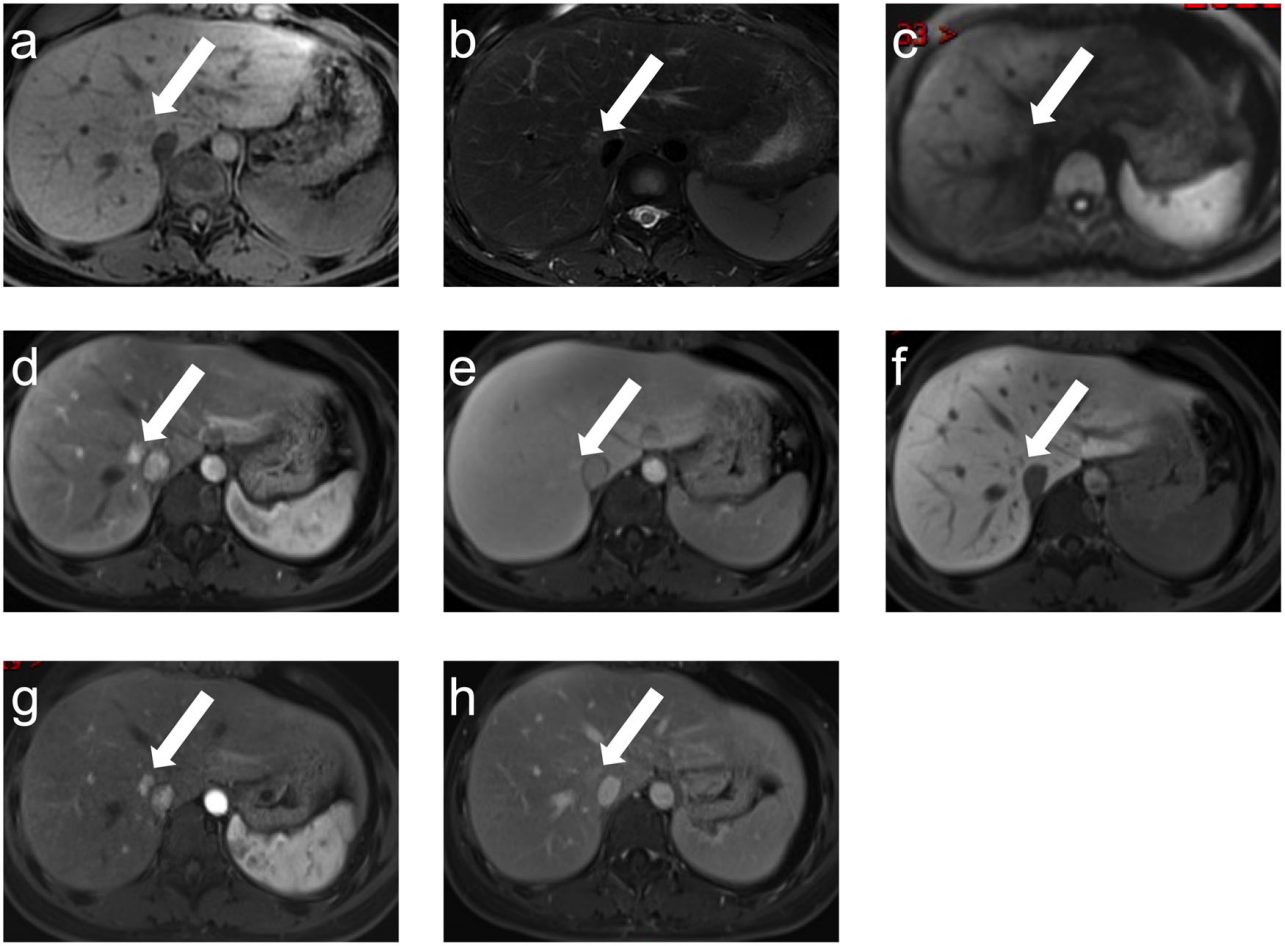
A 28-year-old female with right breast cancer treated with surgery and adjuvant EC-T chemotherapy regimen (6 cycles). A nodule (white arrow) was seen in the hepatic tissue adjacent to the inferior vena cava 42.5 months after the completion of treatment. It showed slightly hypointense on T1WI (a), slightly hyperintense on T2WI (b), isointense on DWI (c), hyperintense on AP image (d), without washout on DP image (e), and slightly hypointense on HBP image (f). After 28.4 months, a decrease in size was seen in AP and DP images (g, h). AP, arterial phases; DP, delayed phases; HBP, hepatobiliary phases
